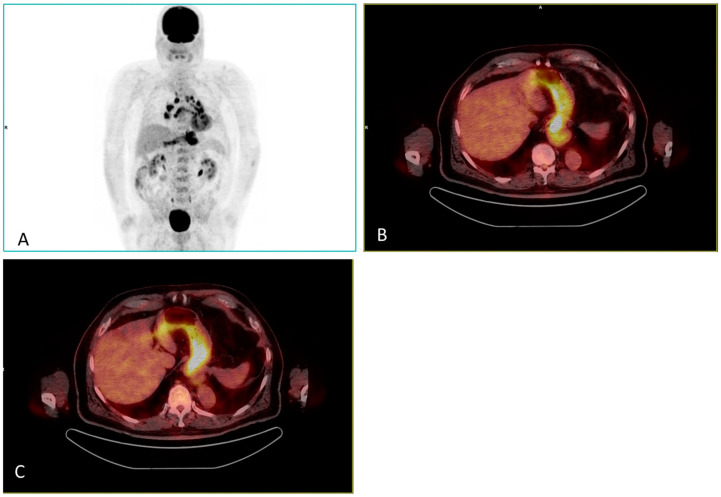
73-year-old man with dorsal melanoma under treatment with Nivolumab and granulomatous reaction sarcoid-like as well as acute gastritis. [^18^F]FDG PET/CT MIP (A) and axial fusion slices (B,C) are presented. Treatment was discontinued.
